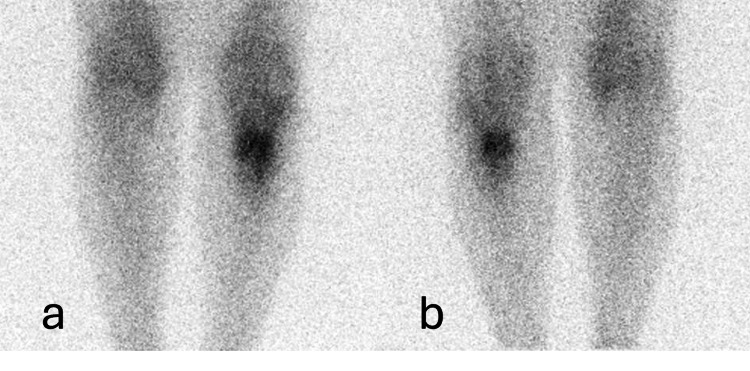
Bone scintigraphy with gallium-67 (a) Anterior view bone scintigraphy with gallium-67 in lower limbs. (b) Posterior view bone scintigraphy with gallium-67 in lower limbs.
